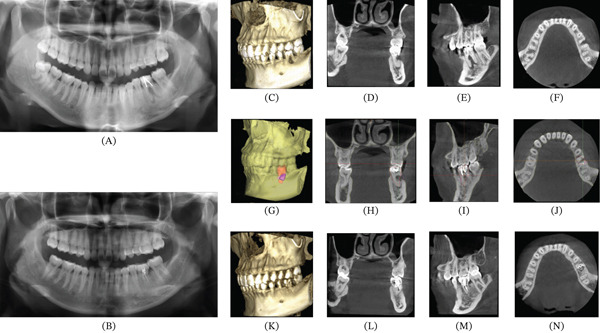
Panoramic radiograph, CBCT and virtual planning of tooth autotransplantation. (A) Preoperative panoramic radiograph; (B) Postoperative panoramic radiograph; (C) Preoperative 3D reconstruction: severe buccal bone loss at #19; (D–F) preoperative CBCT coronal/sagittal/axial views: buccal vertical bone loss and pulpal floor perforation and chronic apical periodontitis at Tooth #19; (G) virtual planning: donor Tooth #1 with bone graft; (H–J) Virtual autotransplantation on CBCT coronal, sagittal, and axial views; the red: Tooth #1, the pink: bone graft; (K) 3D reconstruction at 48 months; (L–N) 48‐month CBCT coronal/sagittal/axial views: buccal bone regeneration and intact lamina dura and continuous PDL space.
